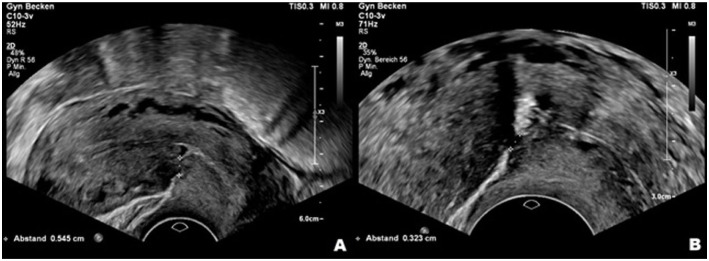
Sonographic evaluation of the residual myometrium thickness before and after contrast medium installation, showing a significant difference in the measured values. (a) Before contrast medium installation. (b) After contrast medium installation.
